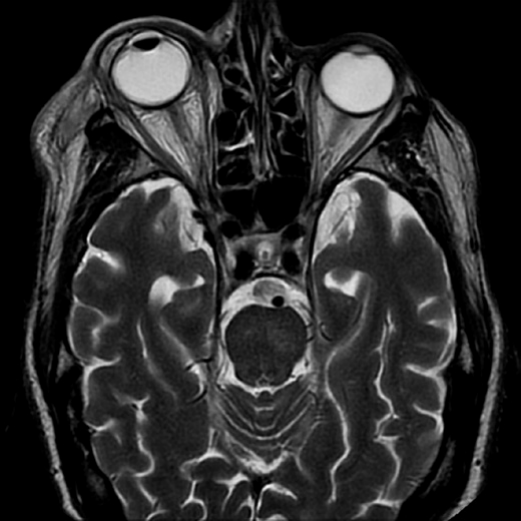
Magnetic resonance image showing proptosis of the right eye and increased
